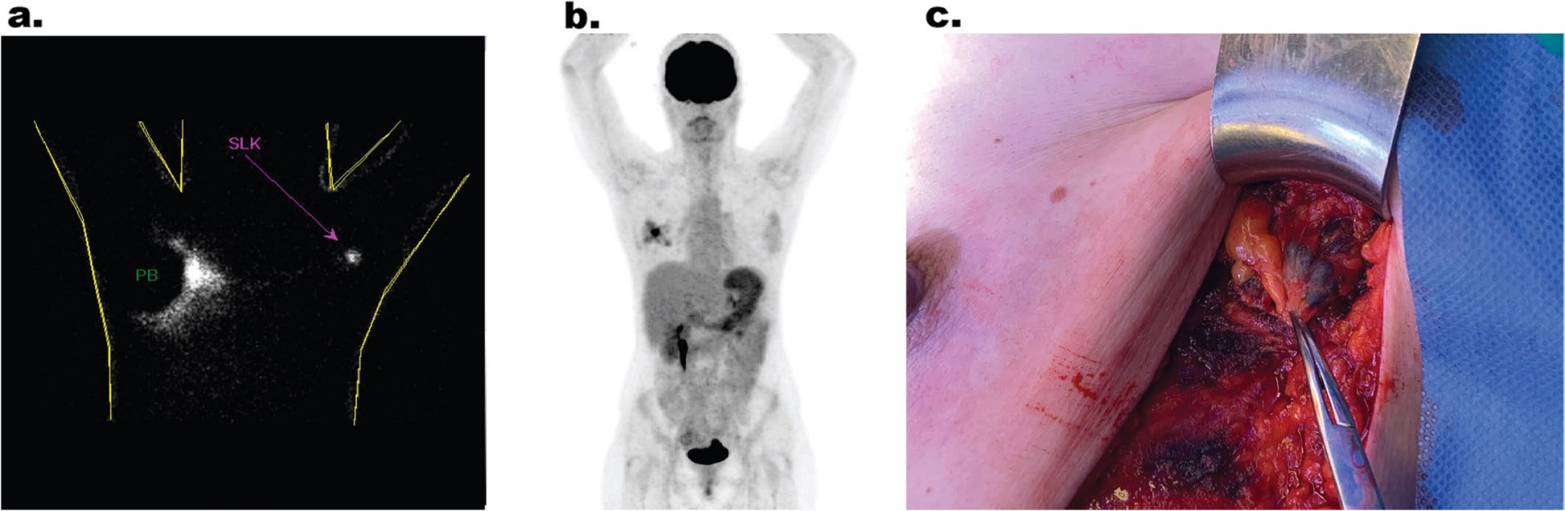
Contralateral lymph node metastasis in recurrent ipsilateral breast cancer. a Planar sentinel node scintigraphy 15 min after periareolar injection of 70 MBq ^99m^Technetium-labelled nanocolloid (NanoHSA-ROTOP®), with detection of the sentinel node (pink arrow) in the left axilla (PB = injection site covered with lead). b Maximum intensity projection of the FDG PET/CT scan, demonstrating diffuse multifocal hypermetabolism of the recurrent mamma carcinoma on the right, without lymph node metastases or distant metastases. c Intraoperative left axilla with detection of two patent blue V and technetium-positive lymph nodes
